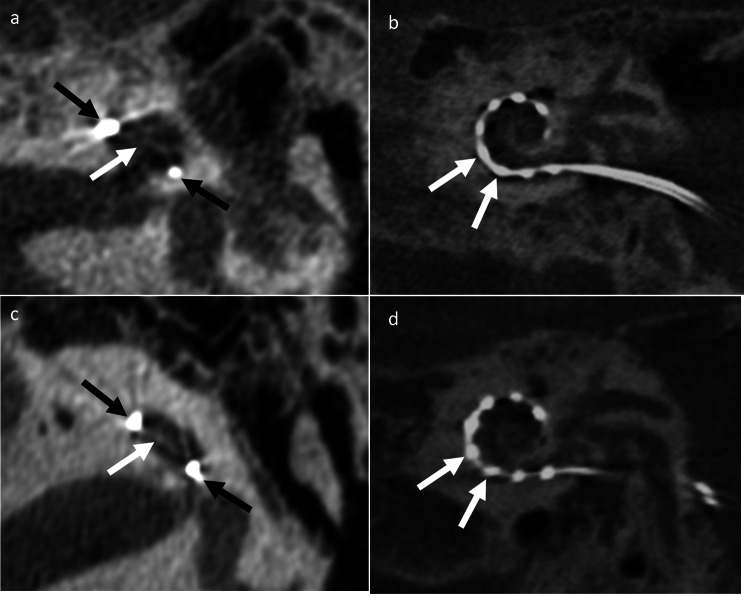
96-kV CBCT (A and B) of a patient with left-sided progressive sensorineural hearing loss and 120-kV (C and D) CBCT of a patient with bilateral congenital surditas (right ear shown) in midmodiolar views and Stenvers views multiplanar reconstructions after insertion of MED-EL Flex 28 electrodes (MED-EL GmbH). 120-kV CBCT provides better ratings compared to 96-kV; in particular, the visualization of the osseous spiral lamina (white arrows) and the depiction of the individual electrode contacts per quadrant (black arrows)
